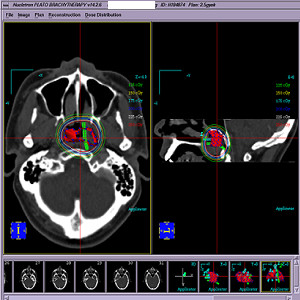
Axial CT image of a NPC patient with stage T2b disease showing four ProGuide Sharp Needles in the parapharyngeal space with 2.5 GY, 2.25 GY, 2.0 GY, 1.75 GY, 1.50 GY, and 1.25 GY isodose lines.
